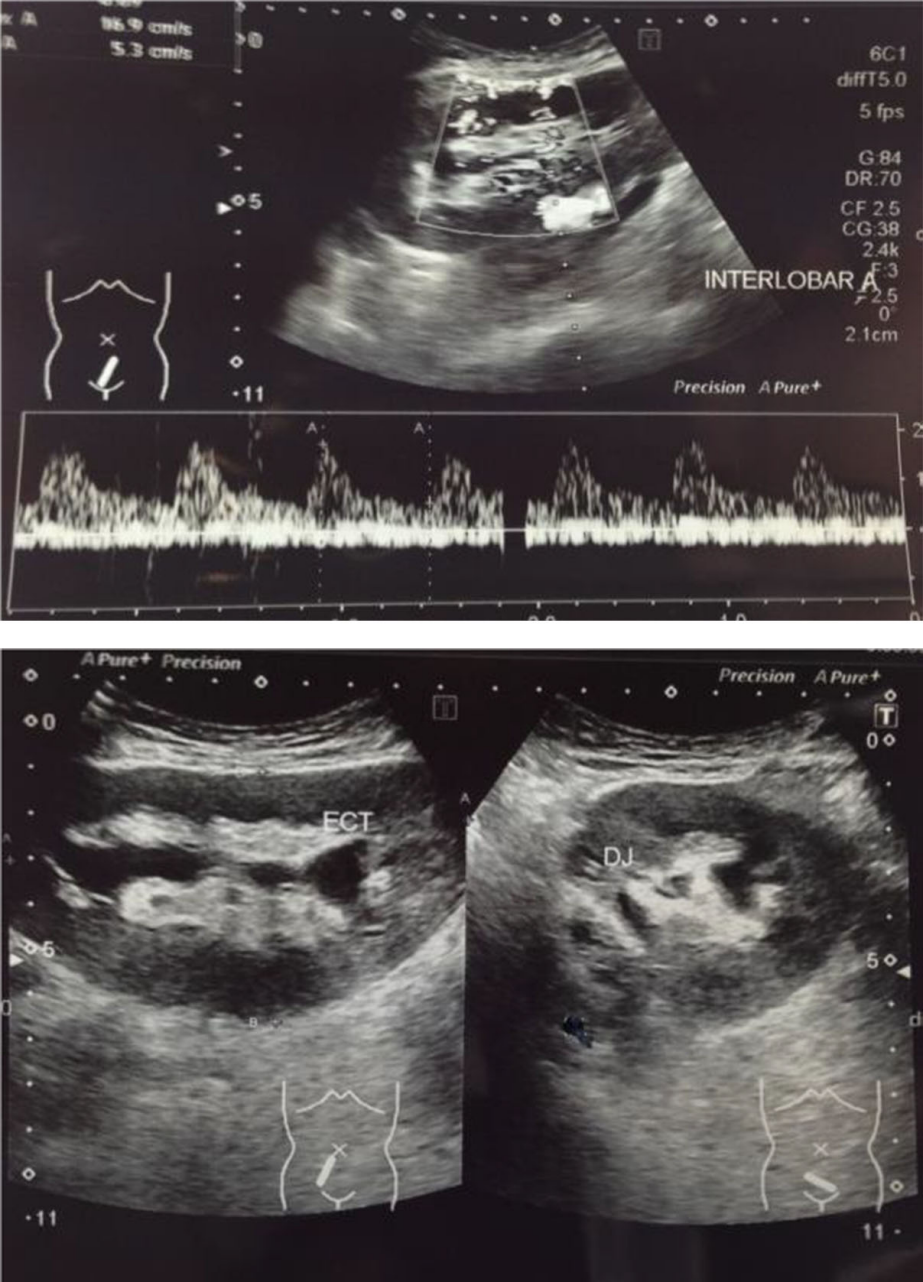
Ultrasound evaluation results of recipient after double-J stent insertion.
